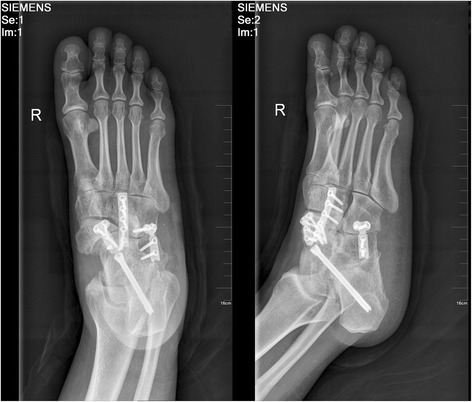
Postoperative radiograph with open triple fusion
